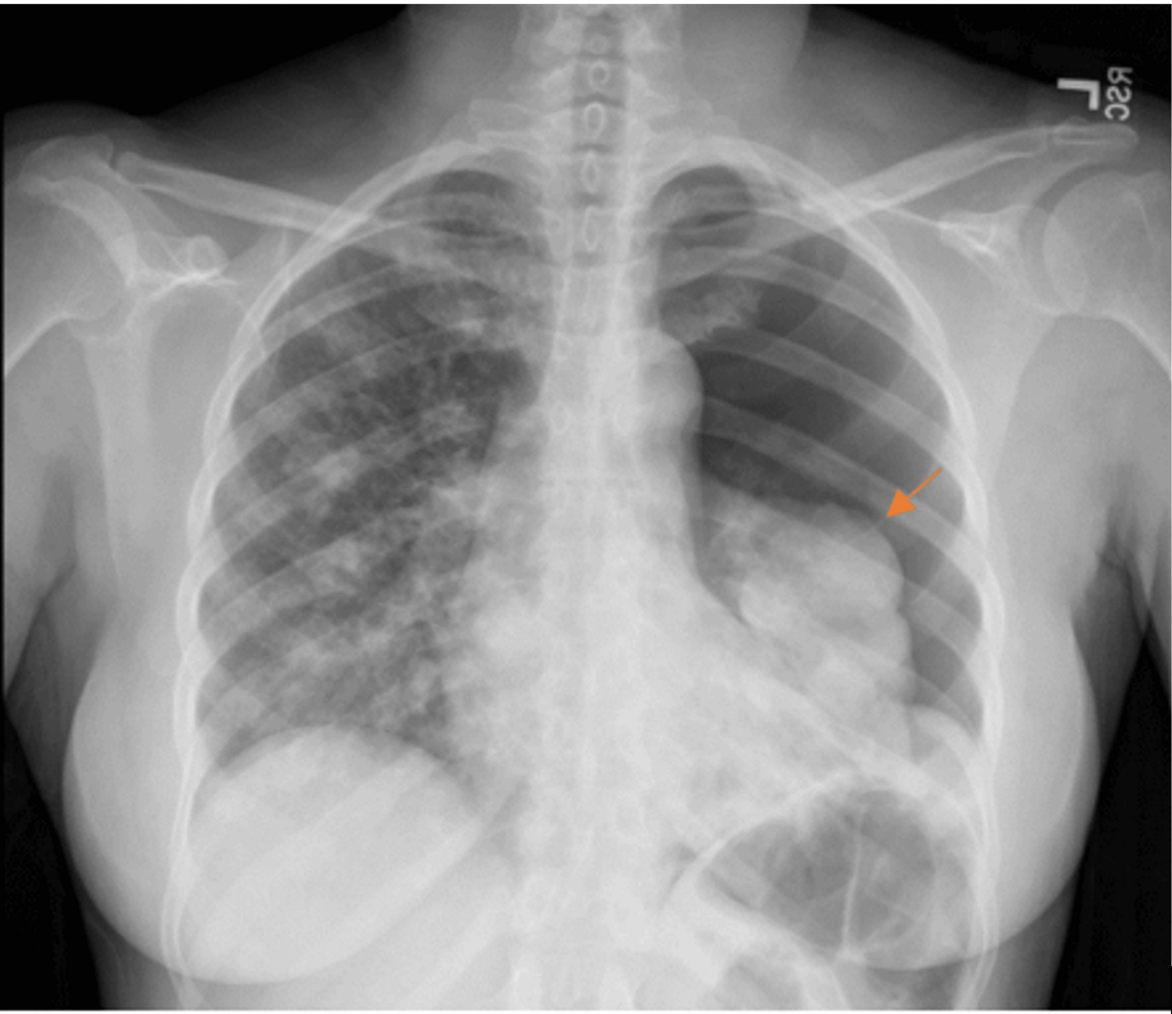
Anteroposterior (AP) chest radiograph demonstrating left PTX and mild right PTX. The orange arrow indicates the site of pulmonary metastasis with overlying PTX in the left lung. PTX: Pneumothorax
